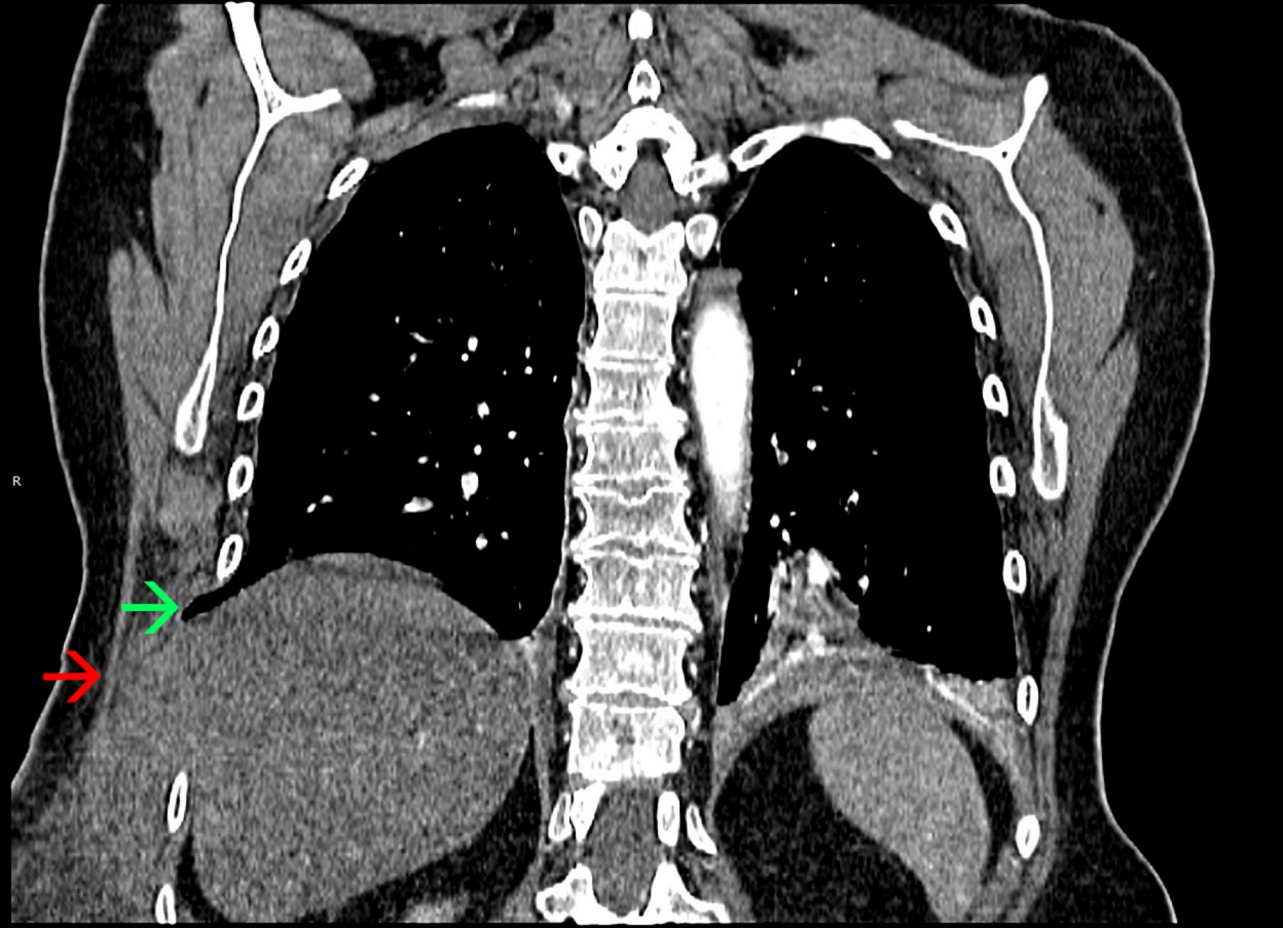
Computed tomography (CT) of the chest. CT scan of the chest revealing a right-sided ninth rib fracture complicated by right lower lung extrusion (green arrow) and liver extrusion (red arrow) between the right eighth and tenth ribs.
